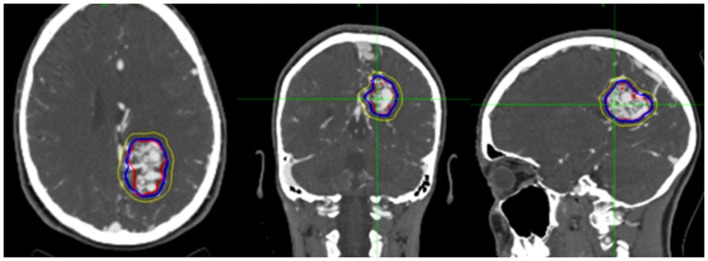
Figure demonstrating treatment planning for a representative case. Planned treatment volume (red), 90% isodose (blue), and 50% isodose (yellow) can be seen in three planes on pre-treatment planning CTA.
